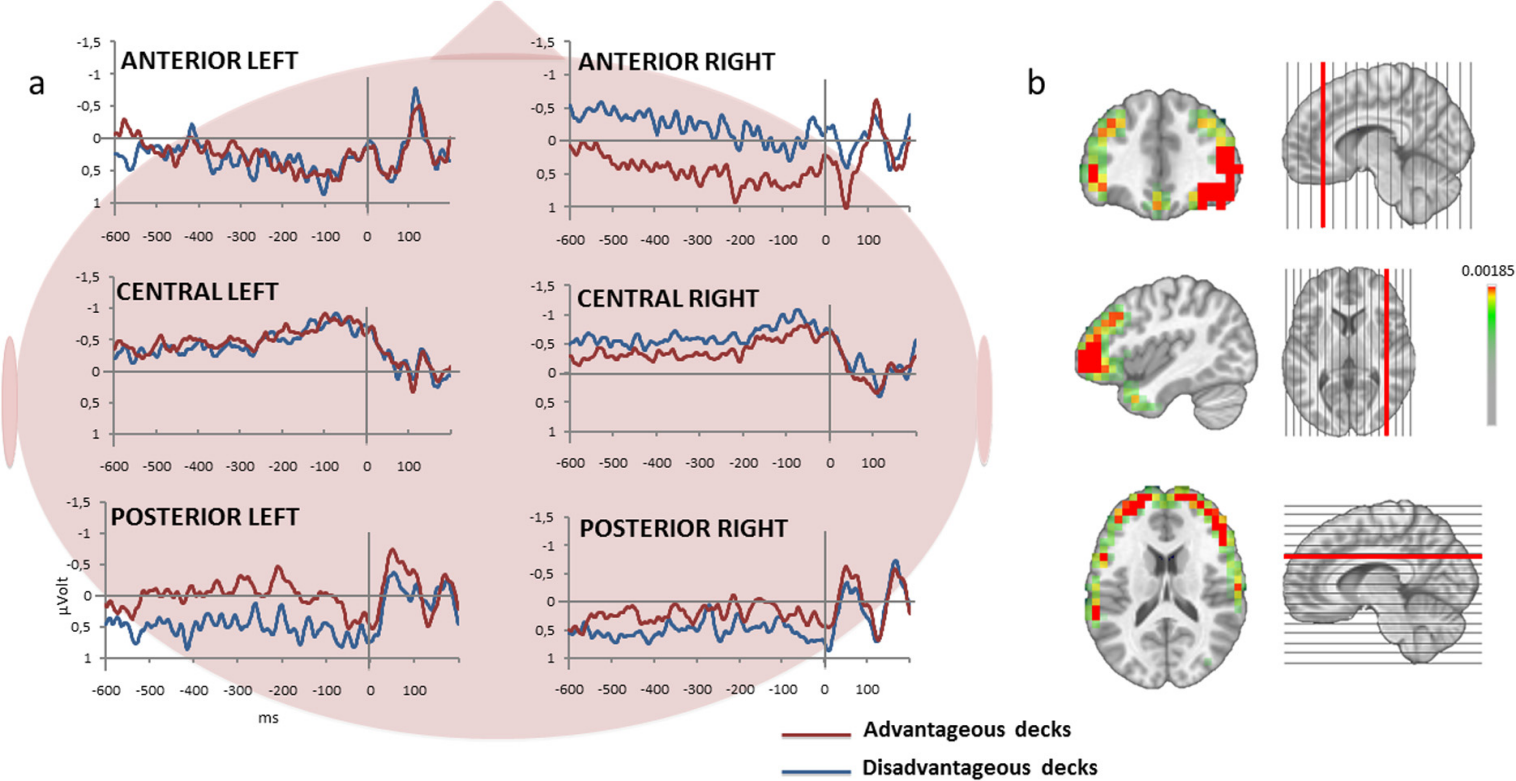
Decision preceding negativity. (a) Grand average of electrophysiological activity of the anticipation of the disadvantageous or advantageous decks on the six clusters. (b) Differences in the anticipation generation between disadvantageous and advantageous decks. A greater activity at the right frontal gyrus level was observed for the disadvantageous decks. Please note that the left side of the brain is shown on the left side of the axial MRI slides.
